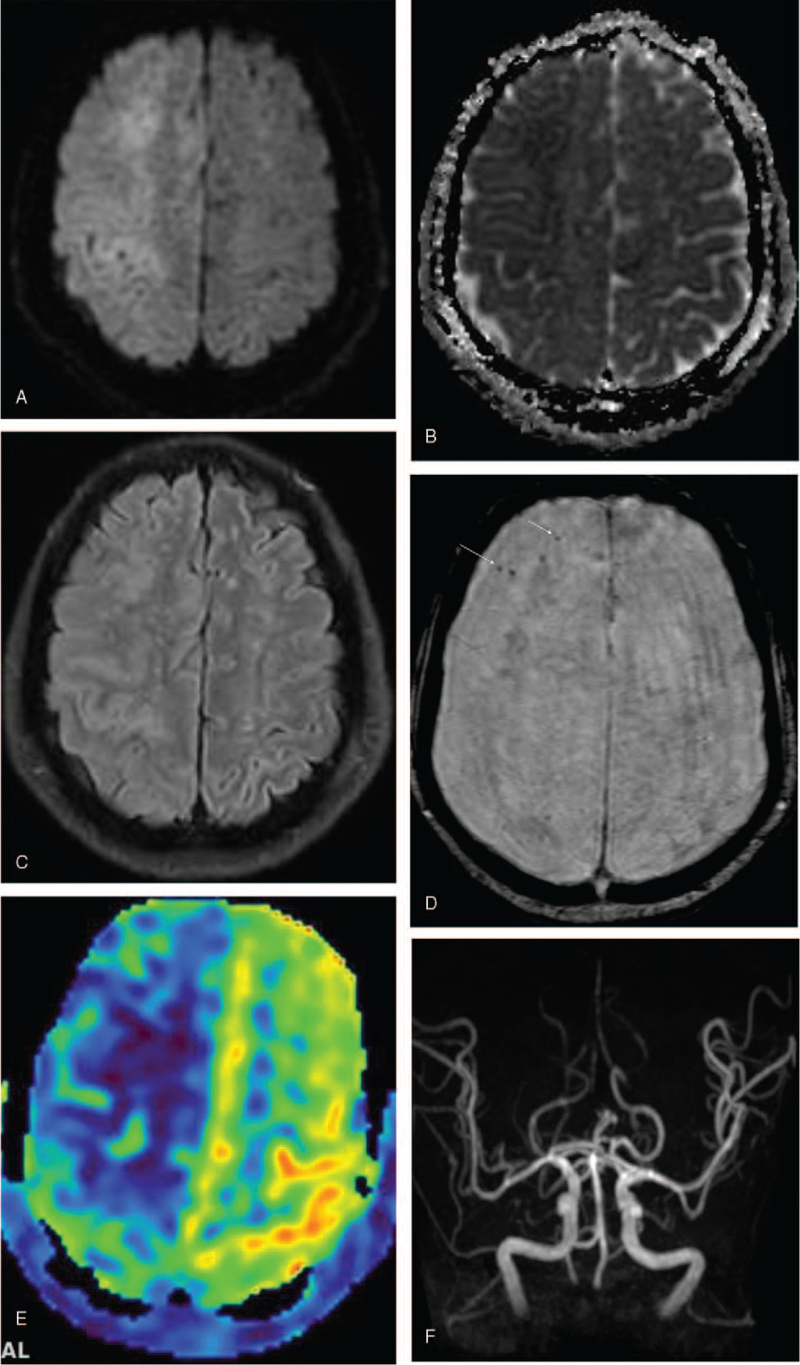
Brain magnetic resonance imaging (MRI) findings. (A) Diffusion-weighted MRI reveals multiple high signal intensities (SI) in the right frontal, right parietal, and left frontal (i.e., superior frontal gyrus) lobes. (B) Apparent diffusion coefficient map shows the corresponding low SI areas. (C) Fluid-attenuated inversion recovery reveals areas of increased SI and cortical swelling, suggestive of acute infarctions. (D) Gradient-echo sequence shows multifocal hypointense blooming dots (arrows) in the right frontal and temporal lobes, consistent with residual air bubbles. (E) Arterial spin-labeling perfusion imaging reveals that the cerebral blood flow is markedly decreased in the right cerebral hemisphere and mildly decreased in the left frontal lobe. (F) Magnetic resonance angiography shows no demonstrable steno-occlusive lesions.
